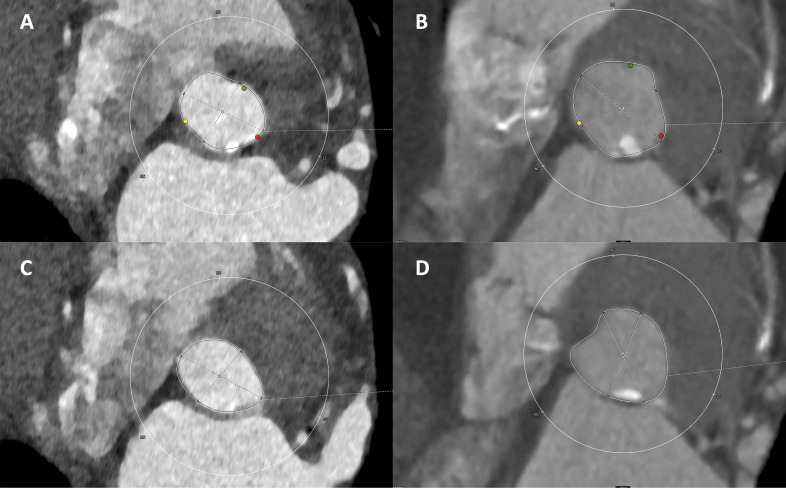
Evaluation of calcification severity at CT angiography. Mild
